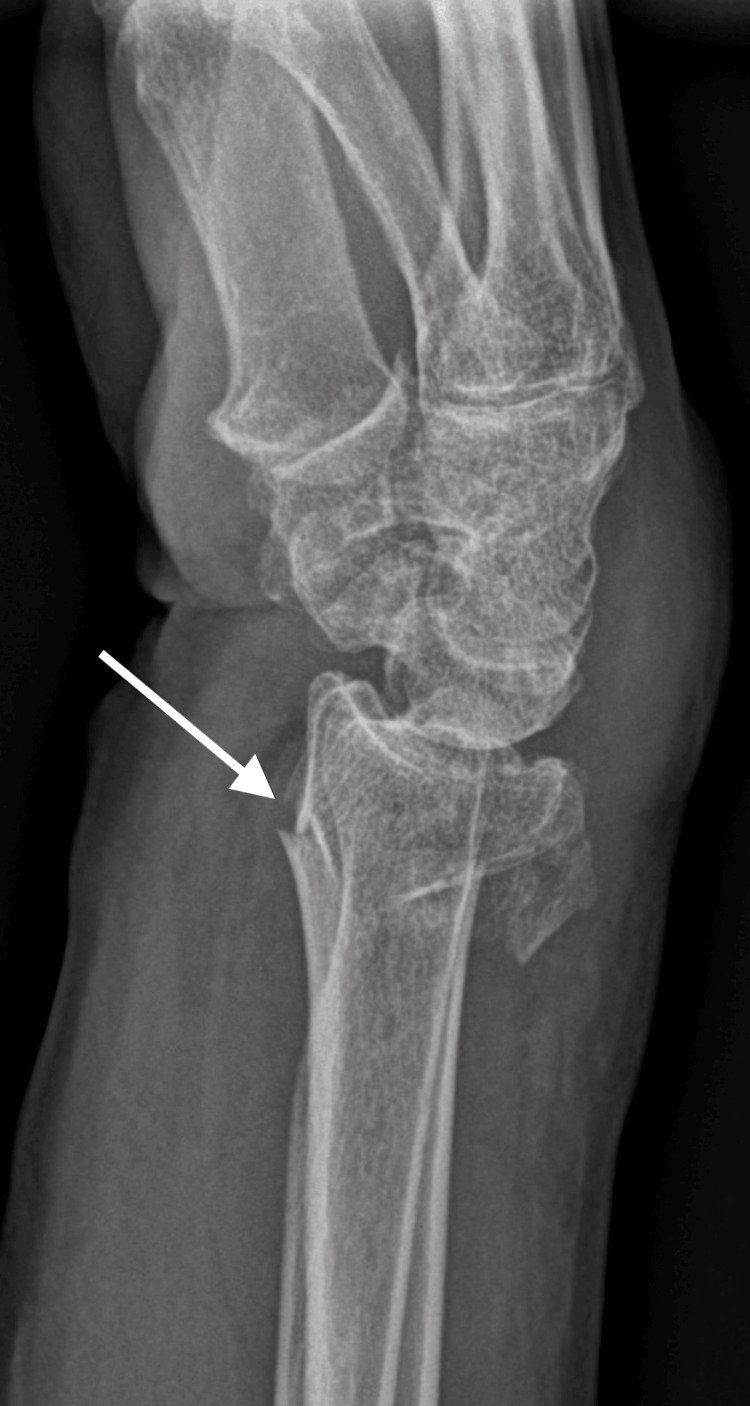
X-ray of initial fracture, lateral view
